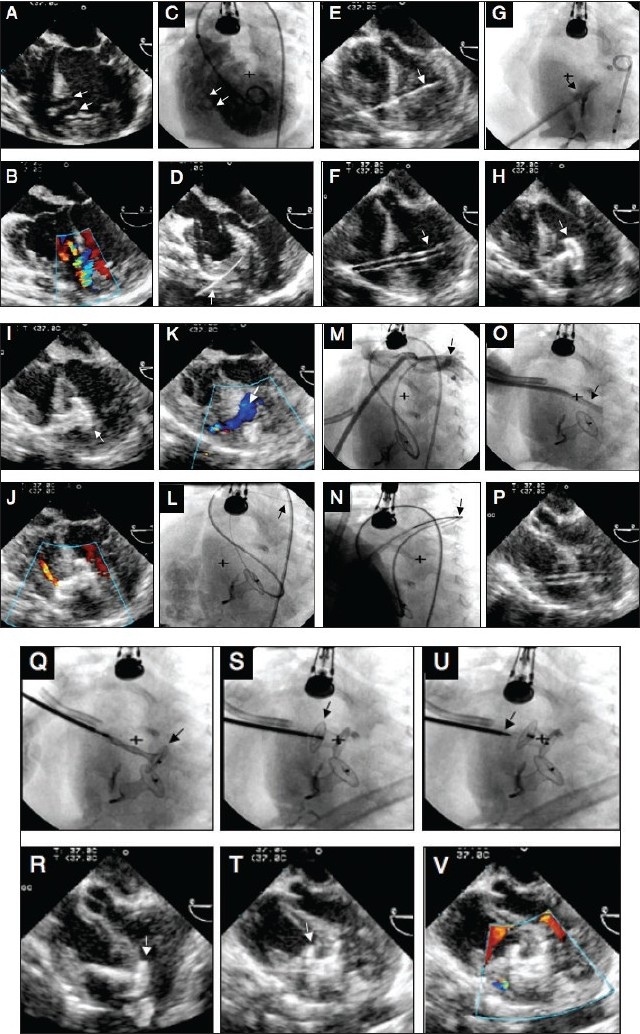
Transesophageal and fluoroscopic images in a 4-month old infant, 5.7 kg child with multiple muscular ventricular septal defects during perventricular device closure of the defects in the hybrid suite. A,B, TEE images without and with color Doppler in 4-chamber view demonstrating the two defects (arrows); C, left ventricular angiogram in the hepatoclavicular projection demonstrating the VSDs (arrows); D, TEE image during passage of the puncture needle (arrow) through the right ventricular free wall; E,F, images during passage of the guide wire and delivery sheath respectively into the LV cavity (arrow); G, angiogram using the sheath showing the tip of the sheath (arrow) in the left ventricle; H, deployment of the disk of the 12-10 mm Amplatzer Duct Occlud (arrow) in the LV cavity; I, deployment of the remainder of the Duct Occlud (arrow); J,K, images after the Duct Occlud has been released, both showing residual shunt (arrow); L, Cine fluoroscopy image during crossing of the residual defect from LV to the left pulmonary artery (arrow) (note the Duct Occlud is in good position); M, hand injection of the sheath that was positioned from the RV free wall to the main pulmonary artery; N, snaring of the wire from the left pulmonary artery (arrow); O,P, delivery sheath is well positioned in the LV cavity by fluoroscopy and TEE (arrow); Q,R, deployment of the LV disk of an 8 mm Amplatzer Muscular VSD device in the left ventricle (arrow); S,T, deployment of the right ventricular disk in the RV (arrow); U, release of the device from the cable (arrow); V, final color Doppler demonstrating no residual shunt.
